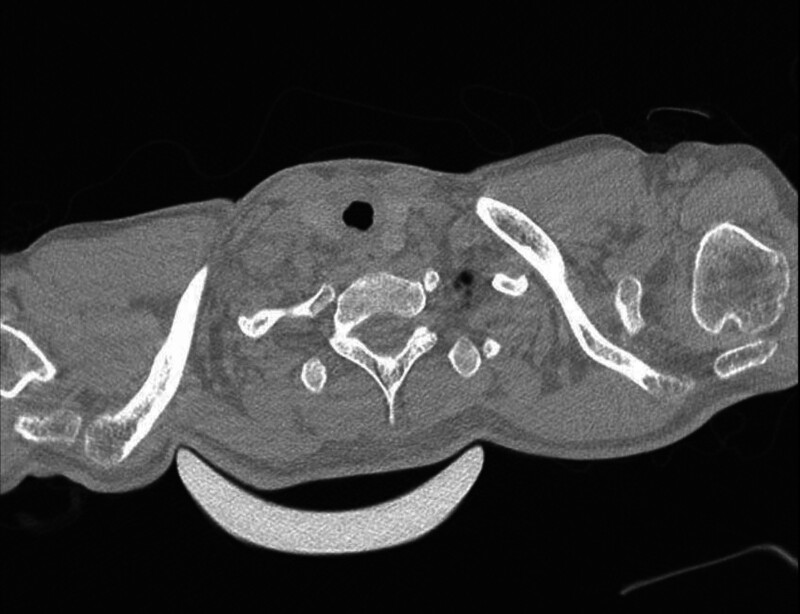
CT image of trachea on the 50th postoperative day. CT = computed tomography.
